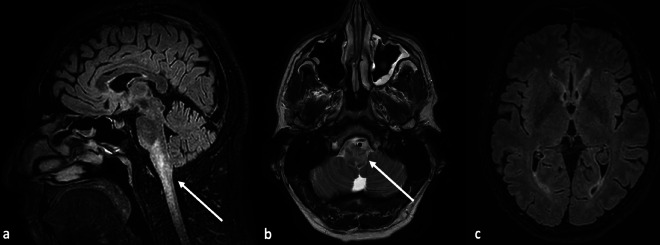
Sagittal FLAIR (a) and axial T2-weighted images (b) showing edematous changes in a swollen brainstem at the level of the pons and medulla oblongata, extending further in the cervical spinal cord (arrows). There was no pathological diffusion restriction or contrast-enhancement (not shown), in keeping with vasogenic edema. Note the absence of edematous changes supratentorially, with a normal appearance of the basal ganglia and subcortical white matter (c).
